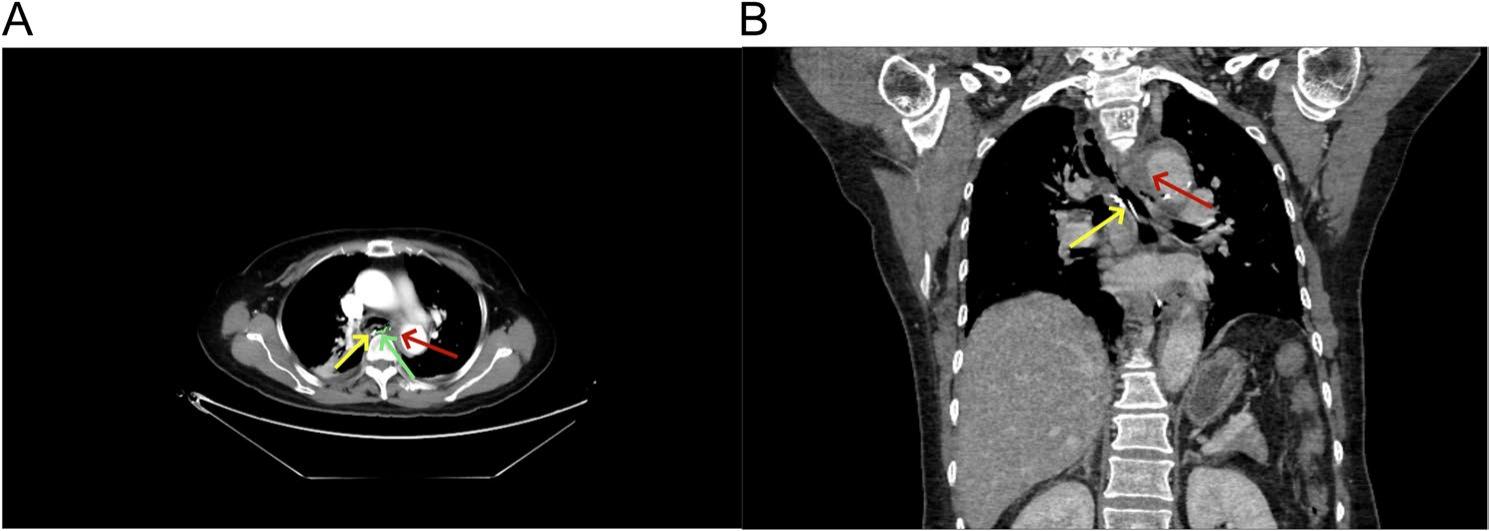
Postoperative Chest computed tomography. *A: Axial CT image; B: Coronal reconstructed CT image; Yellow arrow denotes titanium clip position; red arrow indicates intramural hematoma; green arrow and line delineate the approximate lesion location
